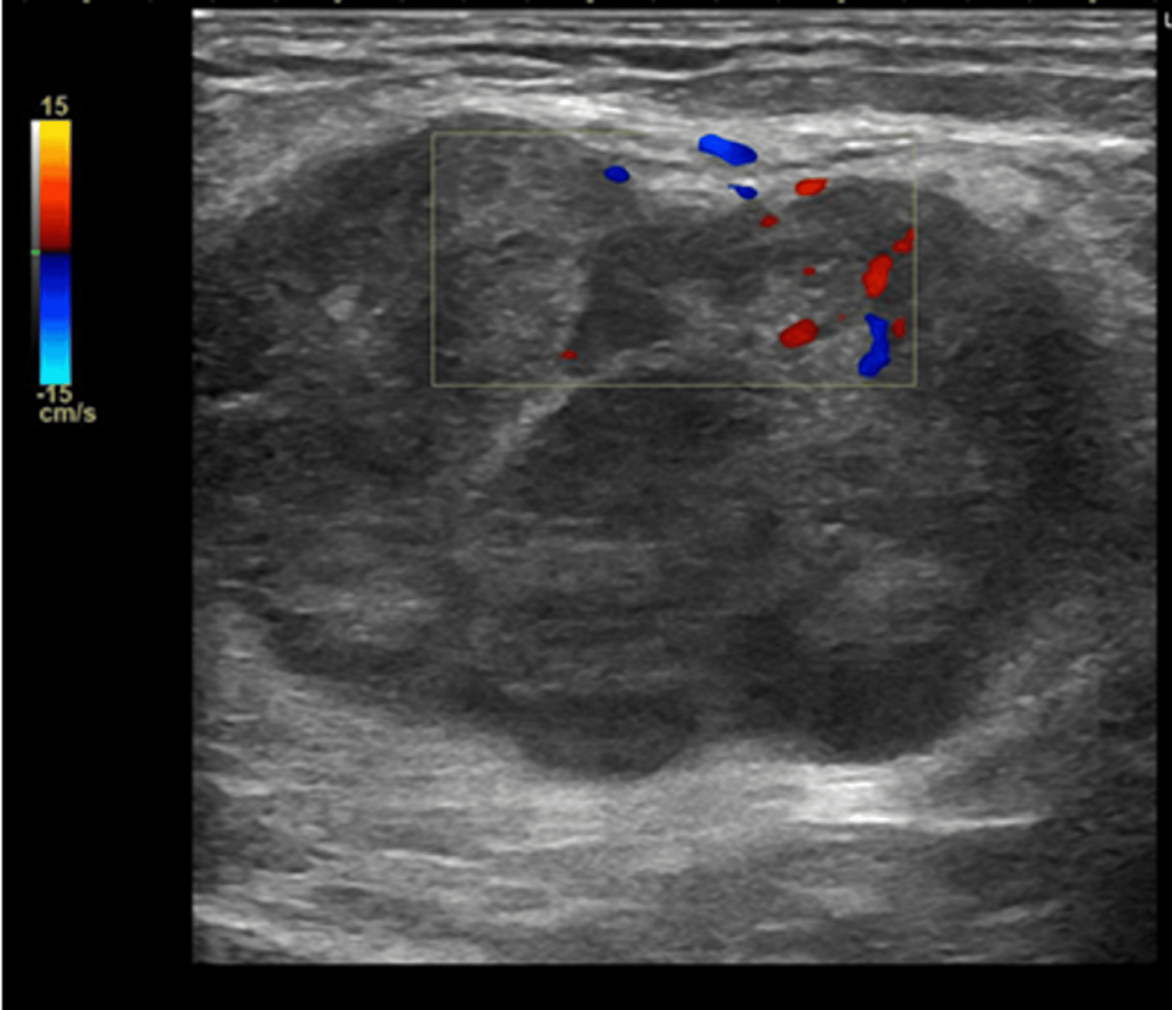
Ultrasonography. A 35 mm hypoechoic mass was observed in the subcutaneous fat layer.
